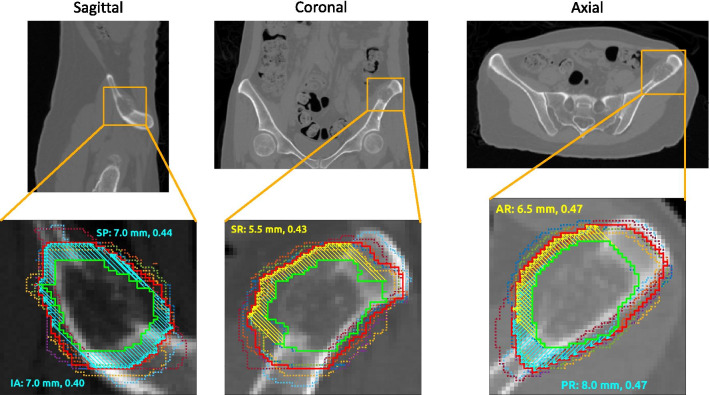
Consensus margin and margin deviation for a metastasis in the iliac crest showing cortical disruption: CT and overlaid contours for a patient with a metastasis in the iliac crest. The GTV and STAPLE CTV are represented by the solid green and red contours, respectively; the expert contours are dotted. The directions of potential intraosseous and extraosseous extension are indicated by the cyan and yellow vectors, respectively. These directions are termed *superior-posterior* (SP), *inferior-anterior* (IA), *superior-right* (SR), *anterior-right* (AR), and *posterior-right* (PR). The text annotations report the consensus margin (mm) followed by the margin deviation (dimensionless) for each direction
